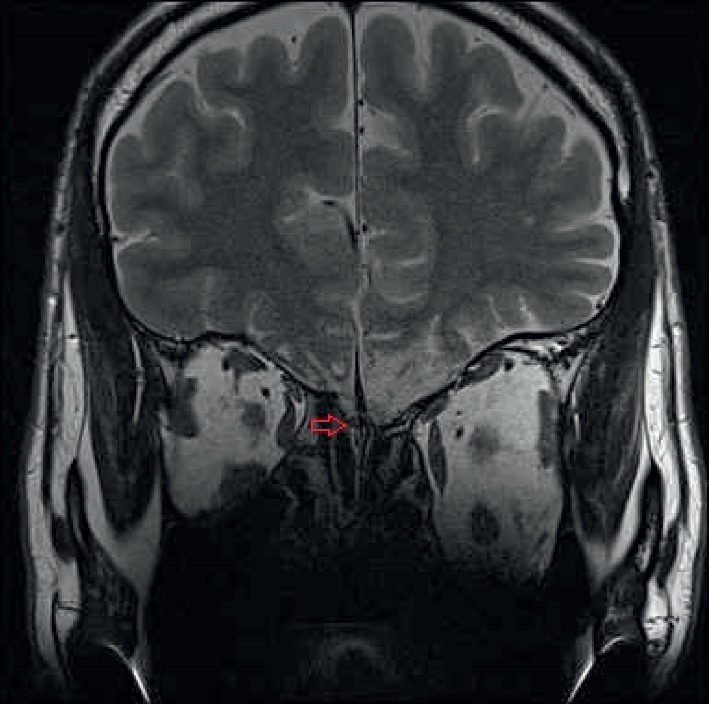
MRI shows that the right olfactory bulb (red arrow) became larger.
